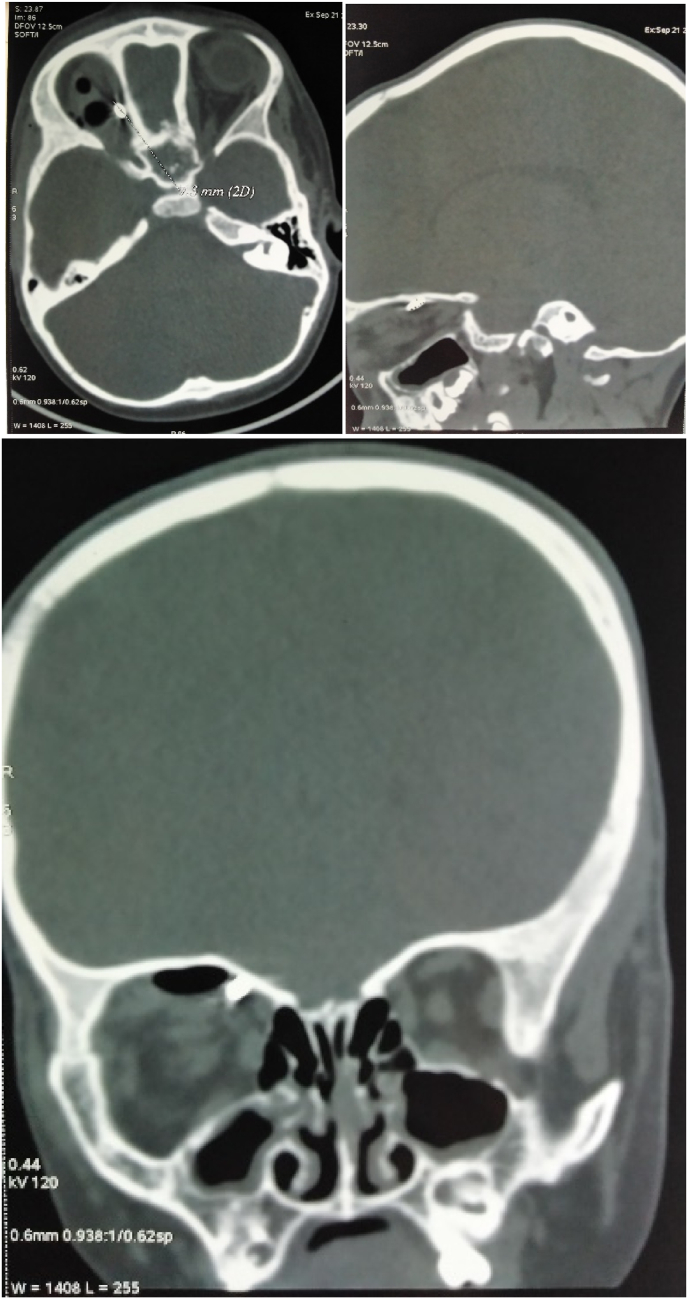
Non-injected CT image in coronal, sagittal and frontal sections showing a metallic foreign body contacting the upper wall of the orbit with pneumobitis.
